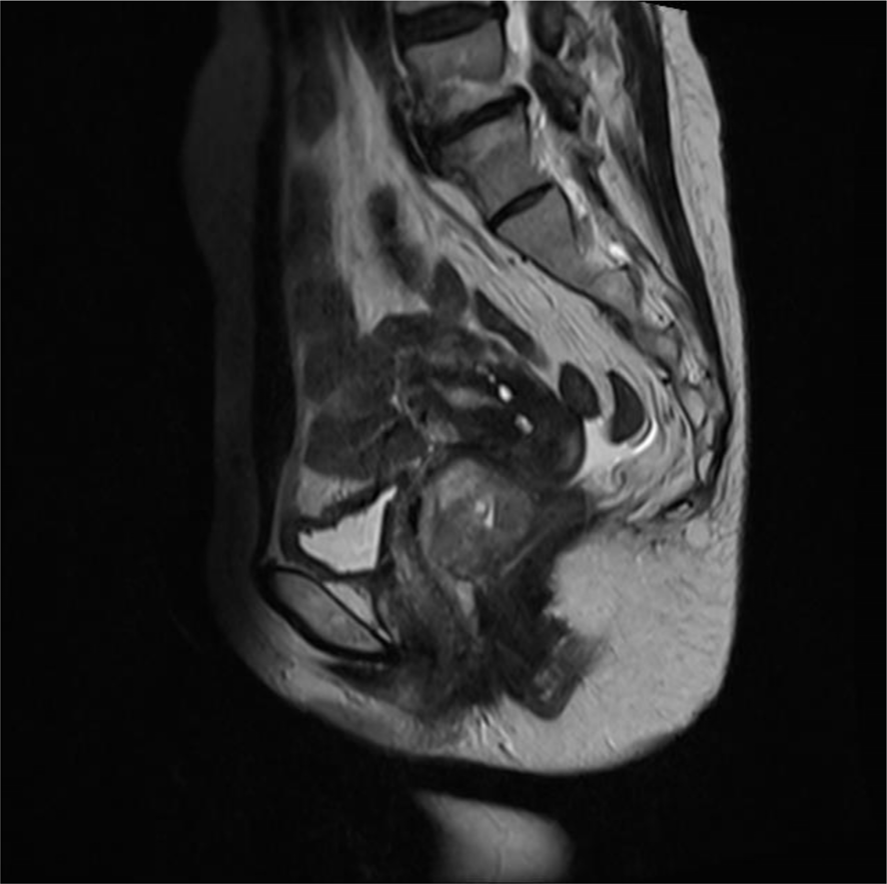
MRI shows a mixed, slightly prolonged T1 and T2 signal mass in the upper segment of the vagina.
